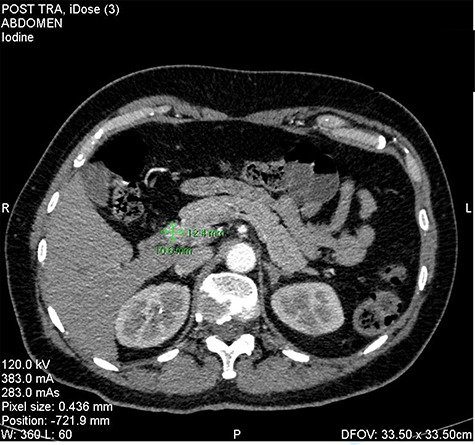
Insulinoma in the head of the pancreas—CT picture in arterial phase (as shown green marks).
